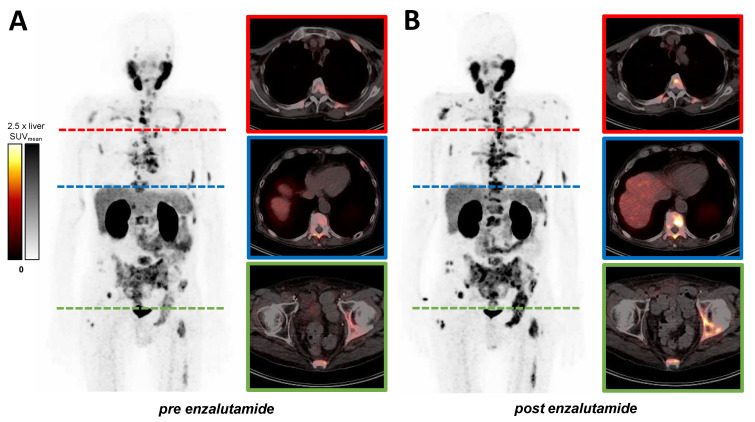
Example of a 68-year-old patient with predominantly bone metastases. Increased uptake can be seen in presented MIP (maximum intensity projection) and exemplary transversal slices of [^68^Ga]Ga-PSMA-11 PET/CT (A) baseline (TLP 3611 mL × SUV, TLP-LR 840 mL × SUV/SUV, TLP-PR 455 mL × SUV/SUV, PSA 408 ng/mL) and (B) after 18 days of enzalutamide medication (TLP 6440 mL × SUV (+78.3%), TLP-LR 1464 mL × SUV/SUV (+74.3%), TLP-PR 688 mL × SUV/SUV (+38.0%), PSA 189 ng/mL (−53.7%)). SUV windowing was set from 0 to 2.5 times SUV_mean_ of the liver.
